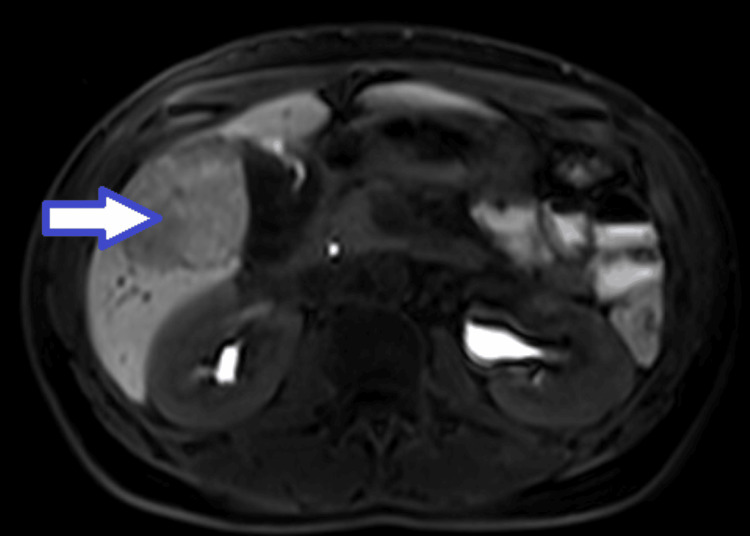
MRI image showing the hepatic nodule The arrow indicates the nodule
